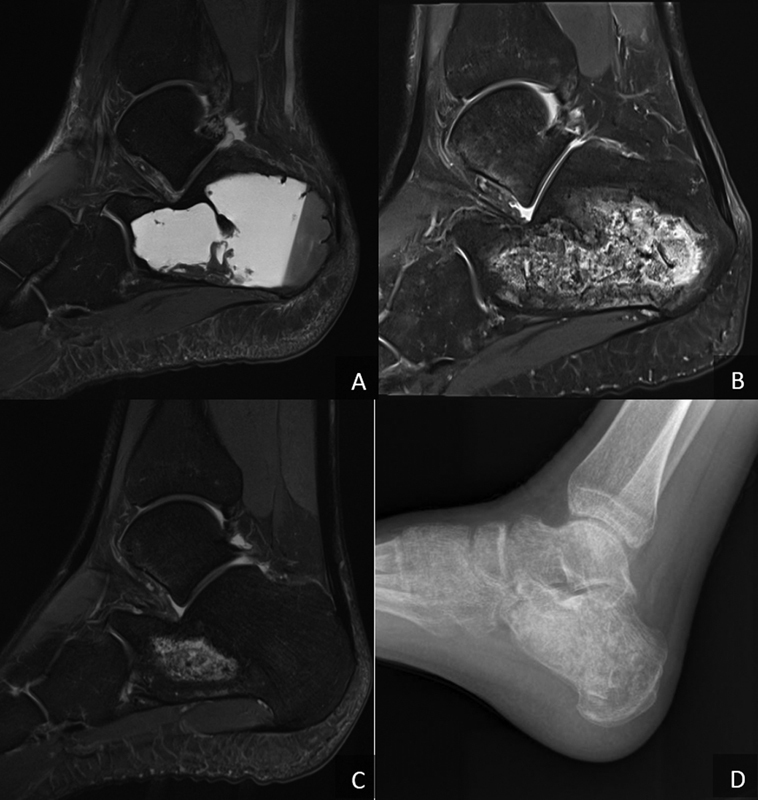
Composition of figures demonstrating magnetic resonance imaging evolution from preoperative (
